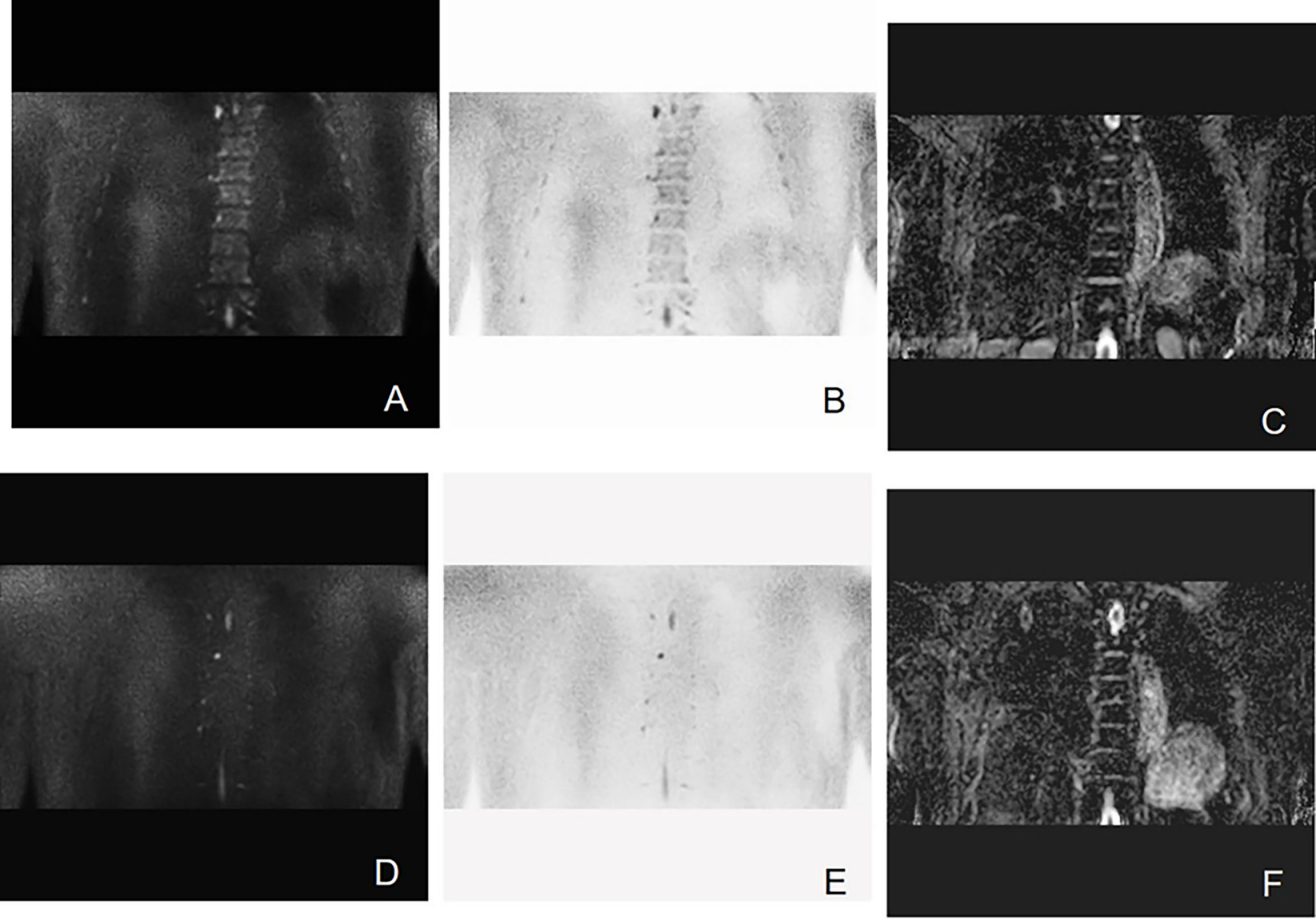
Example of DW-MRI image with treatment response of sCR. A case of 60-year-old man who was diagnosed with MM (type of IgG kappa, RISS Stage II) is inserted here. (A–C) Coronal images of DWI, inverted images, and ADC map at baseline visit. (D–F) Corresponding images of the same patient after three courses of induction chemotherapy (3 VCD + 1 VD). The treatment effect was evaluated as sCR. It has been observed that multiple focal abnormal signals can be seen on the baseline images. However, the number of lesions shown on the images after induction chemotherapy decreased significantly. The mean ADC value of lesions in the scan range also increased slightly from 0.462 × 10^−3^ to 0.570 × 10^−3^ mm^2^/s.
